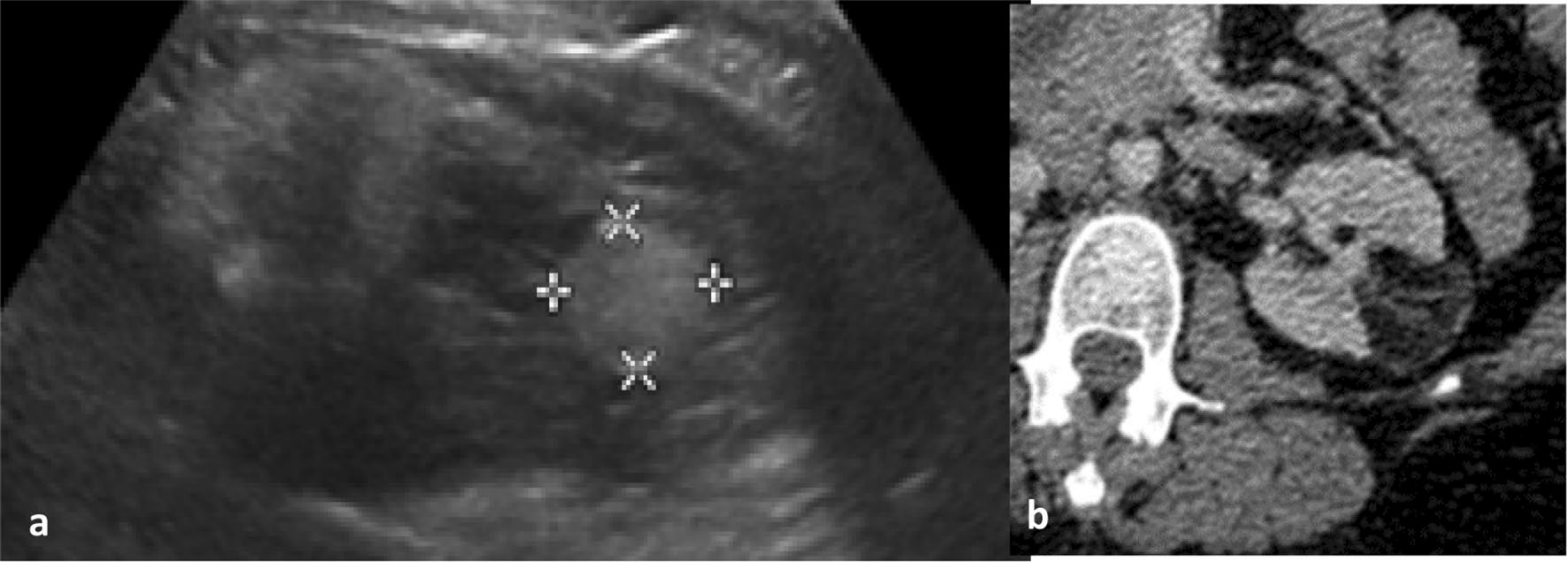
Classical ultrasound (a) and CT (b) appearances of a left renal angiomyolipoma. Solid hyperechoic mass relative to renal parenchyma and of fat attenuation on CT with enhancing components
